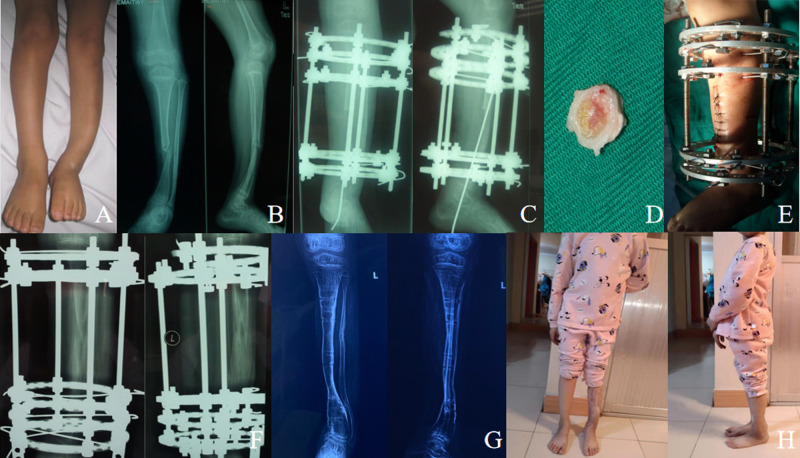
Case of a 6-year-old girl with CPT Crowford type IV associated with NF1. (A) Preoperative photograph showing congenital tibial pseudoarthrosis on the left side and leg length discrepancy (LLD). (B) Anteroposterior and lateral X-rays showing Crawford type IV CPT. (C) Anteroposterior and lateral radiographs viewed 2 days after resection of the pseudoarthrosis and then a Ilizarov fixator combined with intramedullary fixation of the tibia was carried out to manage the pseudarthrosis. (D) Excised fibrous tissue and bone of CPT. (E) Postoperative photos after Ilizarov fixator installation. (F) Anteroposterior and lateral X-ray films at 105 days after operation when docking site was reached; the intramedullary K-wire was removed because of the obvious pin-tract infection in the plantar soft tissue. (G) Anteroposterior and lateral radiographs taken at age 9 years showing a well-aligned tibia and bone union. (H) Function recovery at 41 months after removal of the external fixator.
